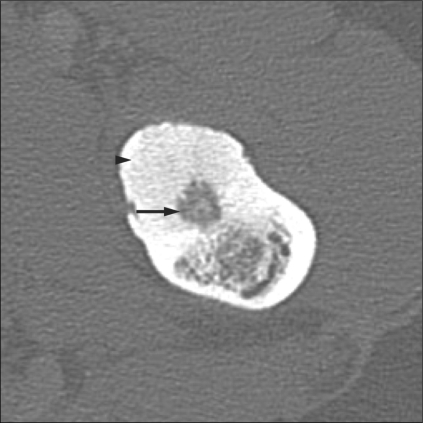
Osteoid osteoma in the femur. Axial CT scan shows a nidus (arrow) within the cortex, with surrounding dense sclerosis (arrowhead)
